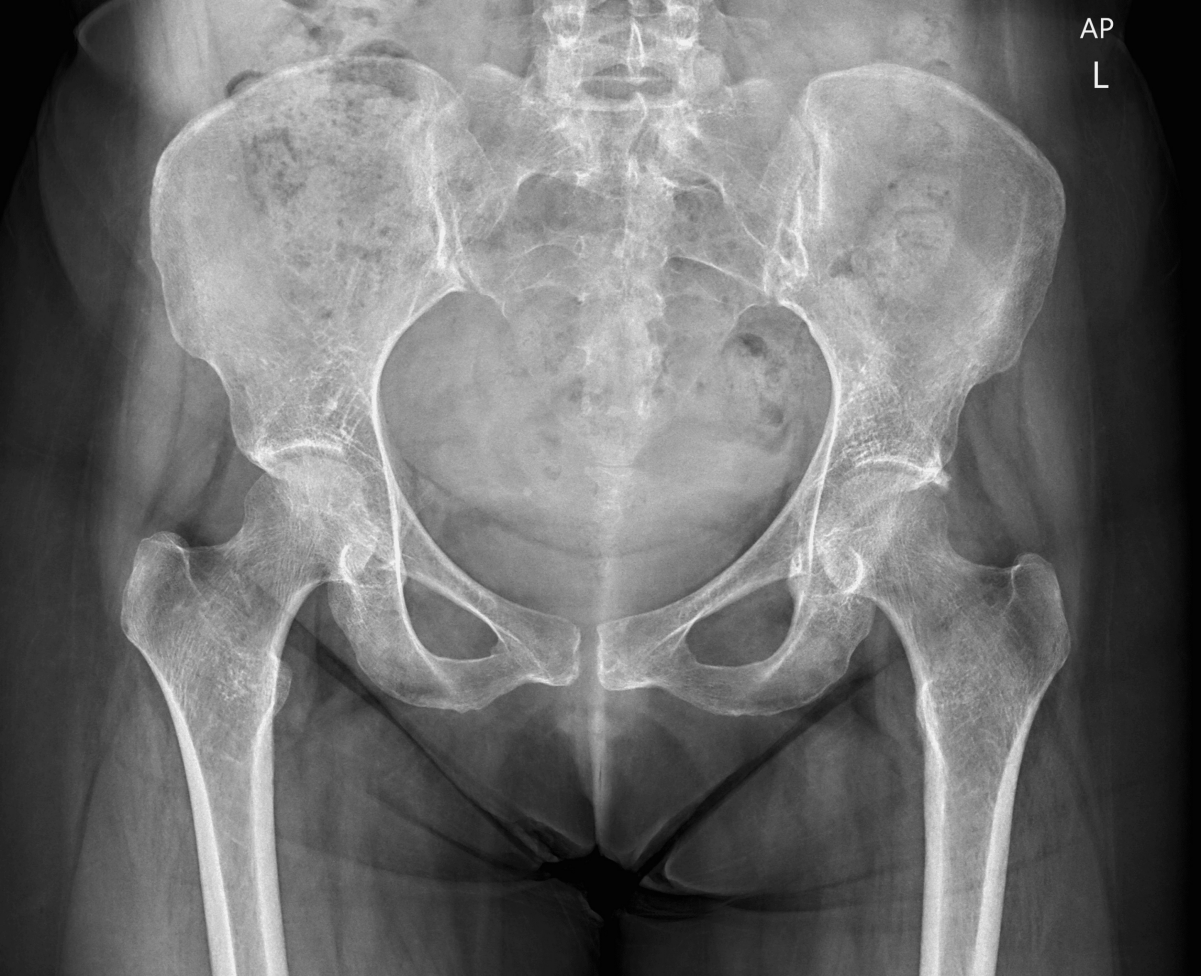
Pelvic X-ray showing no obvious subchondral or subcapital fracture lines
